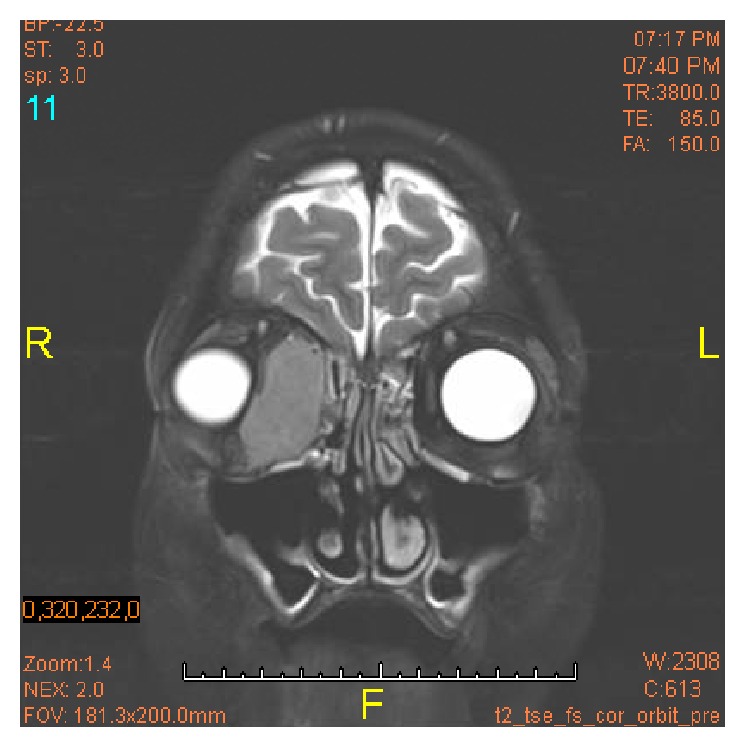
Orbits MRI. Right orbit: there is a 4.1 × 1.9 × 3.5 cm mass in the medial aspect of the right orbit. The mass displaces the medial rectus laterally and the globe anteriorly and laterally. The optic chiasm is normal in appearance. There is no suprasellar or parasellar mass. The pituitary gland is within normal limits in size.
